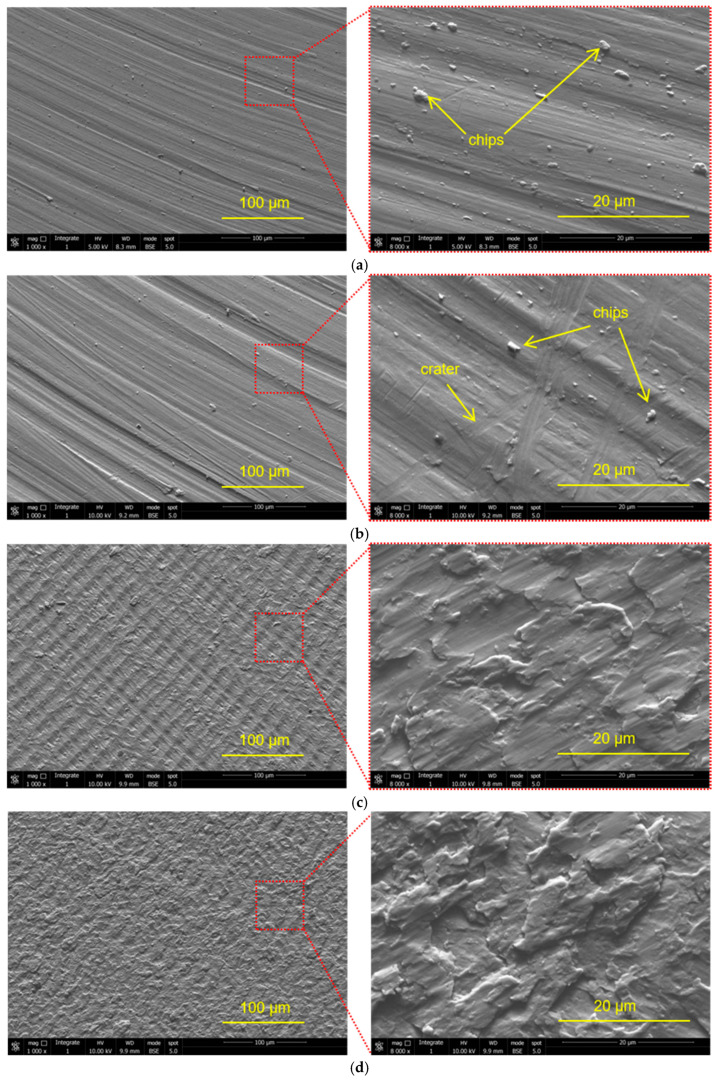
Surface topography after longitudinal ultrasonic vibration milling. (a) A = 0 μm; (b) A = 2 μm; (c) A = 4 μm; (d) A = 6 μm.
